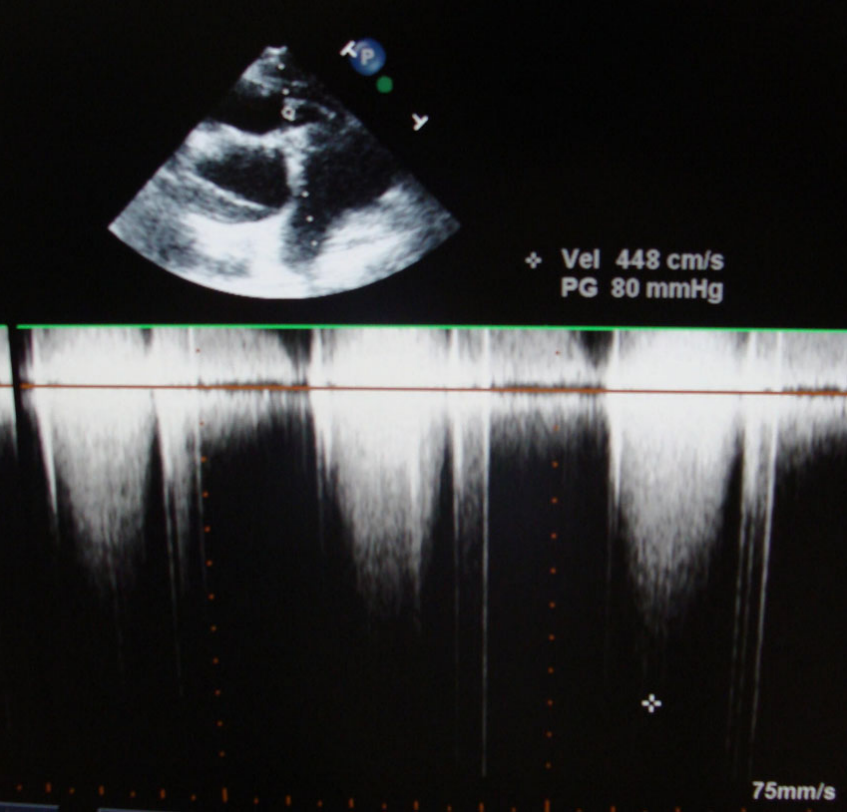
Increased pressure gradient (maximal pressure gradient of 80 mmHg) of the Contegra valve assessed by continuous wave Doppler in short-axis parasternal view of transthoracic echocardiography.
